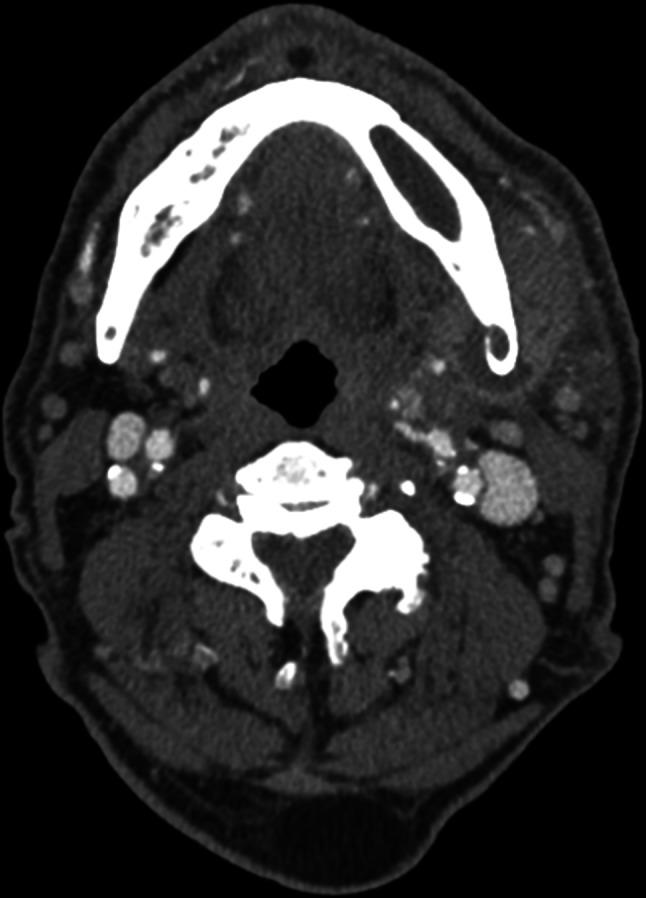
CT image of the neck demonstrating sclerotic lytic lesions of the left mandible, and a 2.6×1.7×1.6 cm hypodense peripherally enhancing collection surrounding the left posterior mandibular body with surrounding oedema.
